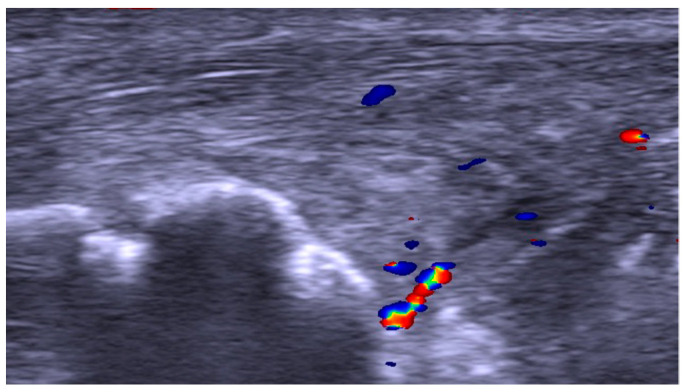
Ultrasound and colour Doppler image showing enlarged retro-calcaneal bursa with localised high blood flow (colour), thickened tendinopathic distal Achilles tendon, and Haglund-like deformity of the upper calcaneus.
